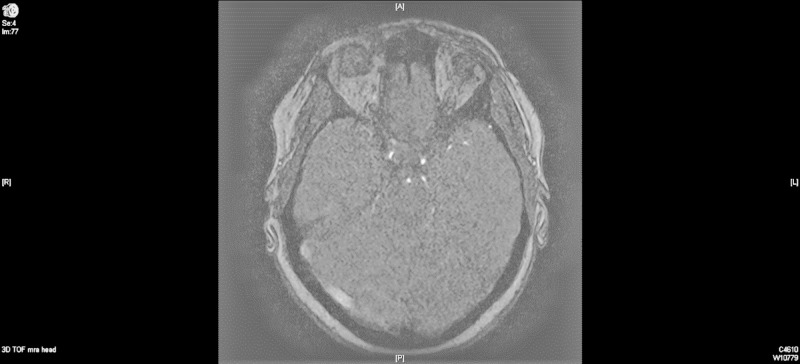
Magnetic resonance imaging (MRI) angiogram brain without contrast, day 1.
